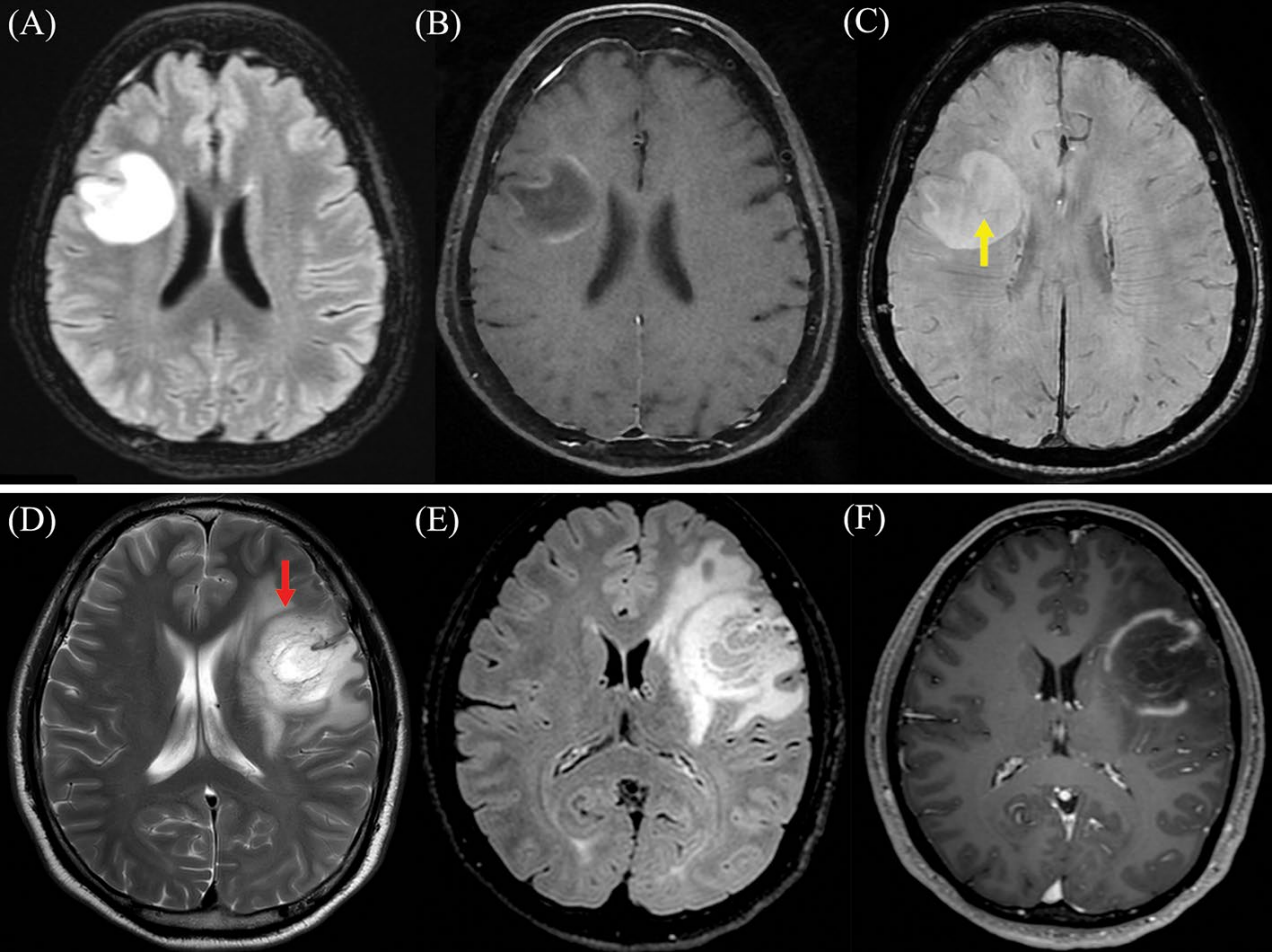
Representative brain magnetic resonance imaging (MRI) Patient 12: (A) Axial T2-weight Fluid-Attenuated Inversion Recovery (FLAIR), (B) Axial Post-gadolinium T1-weighted imaging, and (C) Axial T2 star-weighted angiography (SWAN) reveal the presence of the central vein sign (CVS), denoted by the yellow arrow. The CVS is characterized by a hypointense thin line or small dot centrally situated within the lesion. Patient 2: (D) Axial T2-weight imaging, (E) Axial T2-weight FLAIR, and (F) Axial Post-gadolinium T1-weighted imaging exhibit the T2-weighted hypointense rim, highlighted by the red arrow. The T2W hypointense rim is defined by a thin border of T2-weighted hypointensity, contrasting with the hyperintense regions of the lesion's core and the surrounding edema.
